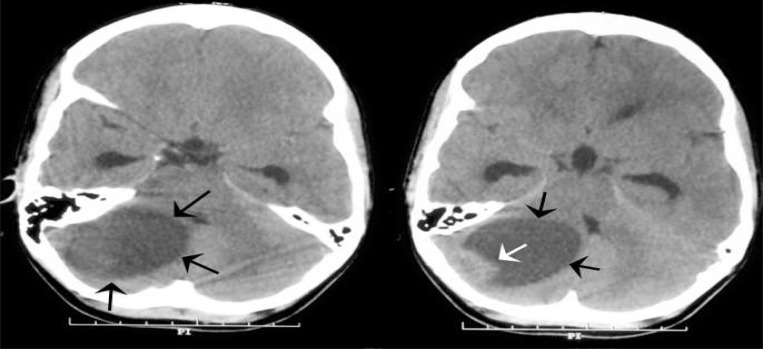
Brain CT scan of the patient indicating a cystic lesion in right cerebellum (black arrows) with mural nodule (white arrow)
